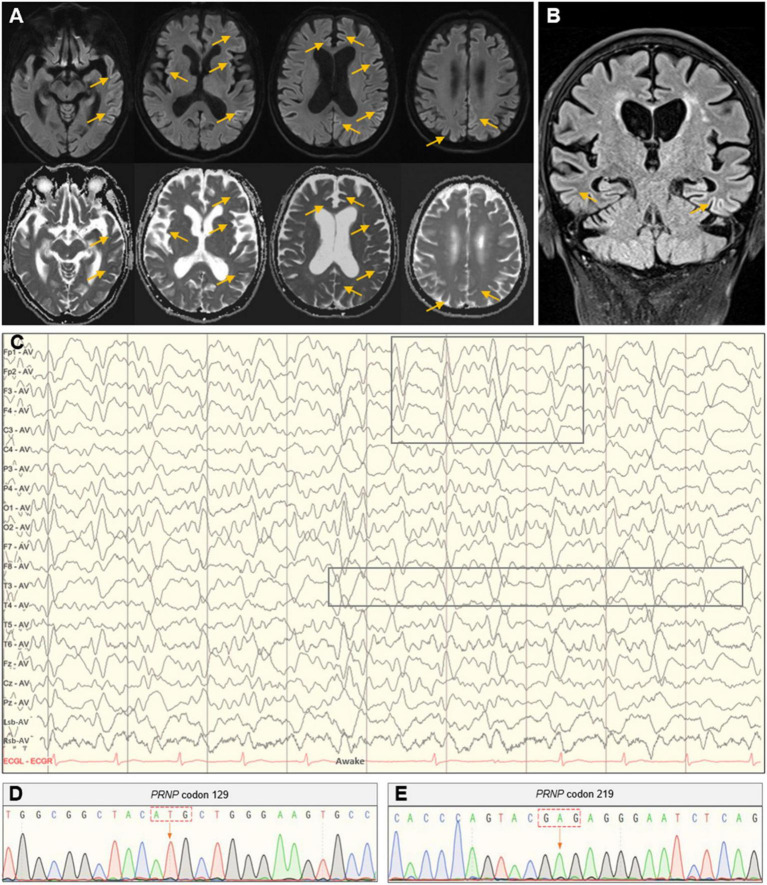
Head MRI, EEG, and *PRNP* gene analysis. (A) Axial imaging showed hyperintense signals in the bilateral parietal-occipital and frontal lobes in the DWI with correlating hypointense signals in the ADC map. (B) Coronal imaging showed hyperintensities in the bilateral parietal-temporal lobes in the FLAIR sequence. (C) The PSWC on EEG. (D,E) Polymorphic analysis of the *PRNP* gene at codon 129 and 219.
